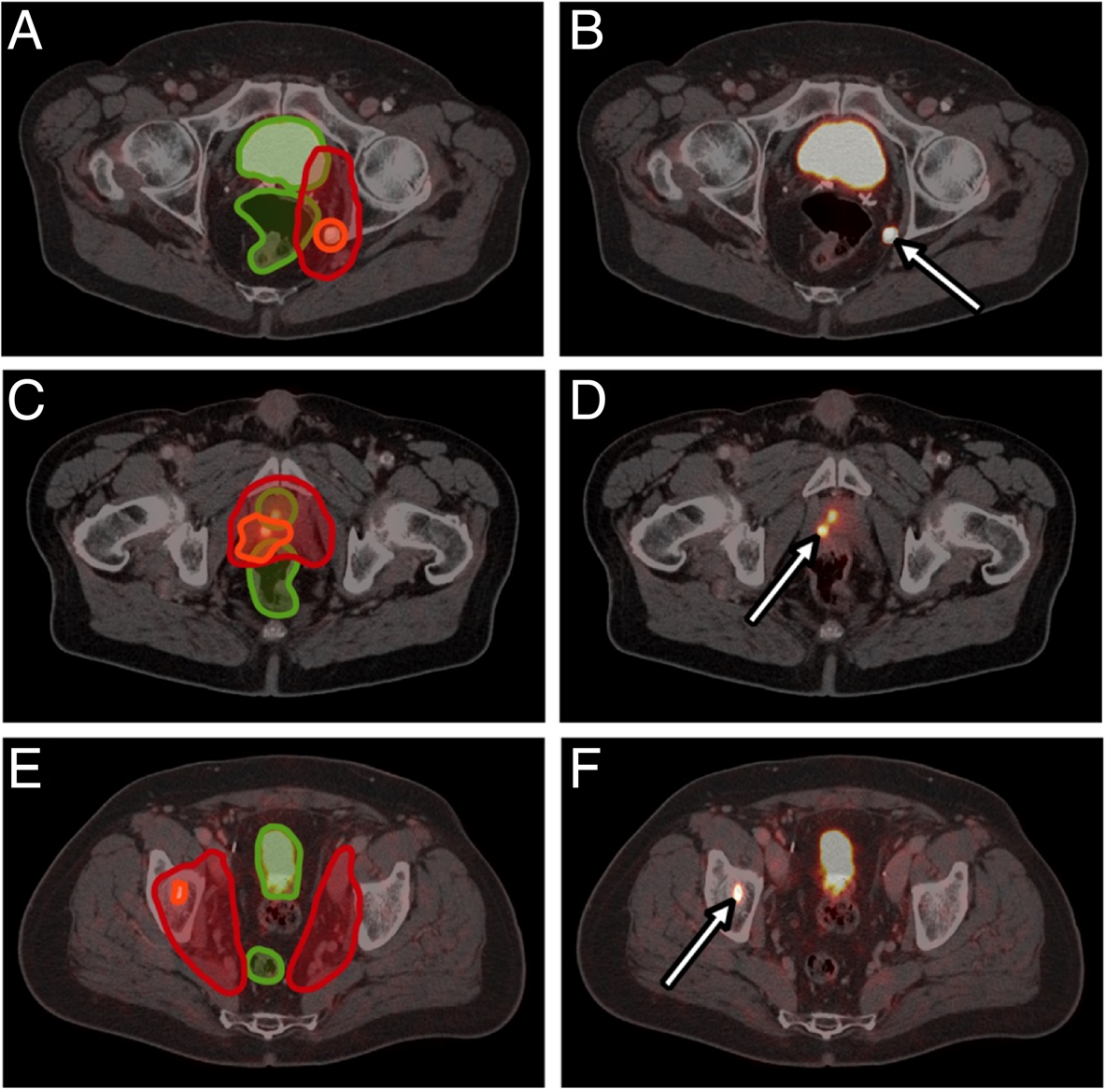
Radiotherapy treatment planning in three patients based on PSMA PET/CT information. ^68^Ga-PSMA PET/CT (B, D, F) and target volumes with simultaneously integrated boost volumes (A, C, E) are shown. Figs. A and B show a patient with PSA recurrence and evidence of lymph node metastasis in ^68^Ga-PSMA PET/CT who was treated with a simultaneously integrated boost to the lymph node. Figs. C and D show a patient with PSA recurrence due to local relapse in the region of the former prostate gland. This local relapse was treated with a simultaneously integrated boost volume. Figs. F and E show a patient with singular osseous metastasis that was treated with a simultaneously integrated boost
